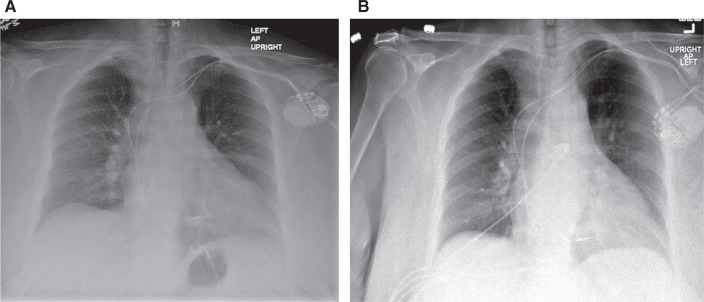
A: Chest X-ray at implant with atrial and ventricular leads in place. B: Chest X-ray showing atrial lead dislodgment that occurred a few days after device implant.
